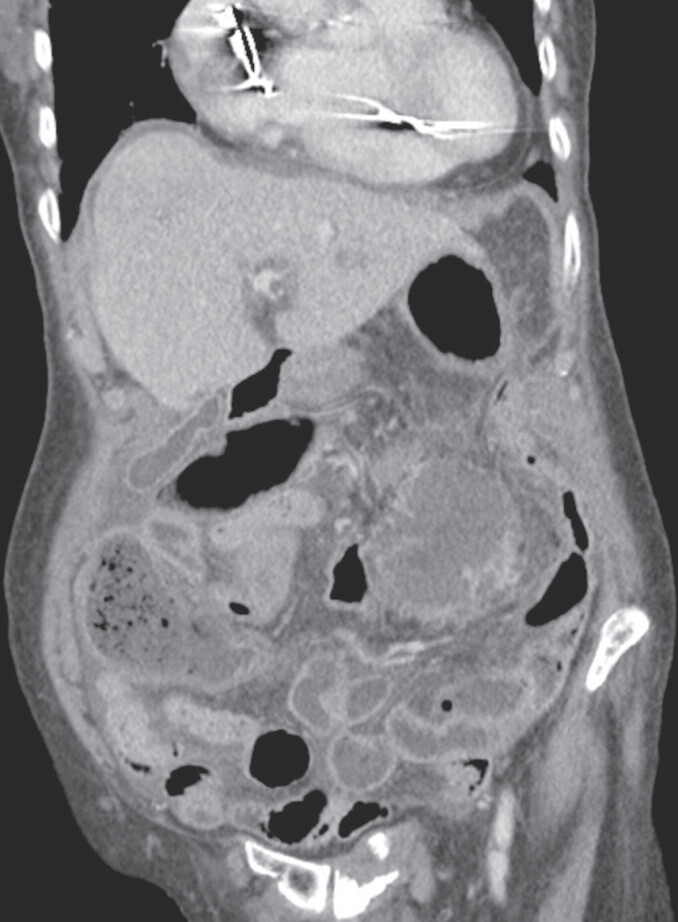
Abdominal computed tomography demonstrating a perforated
